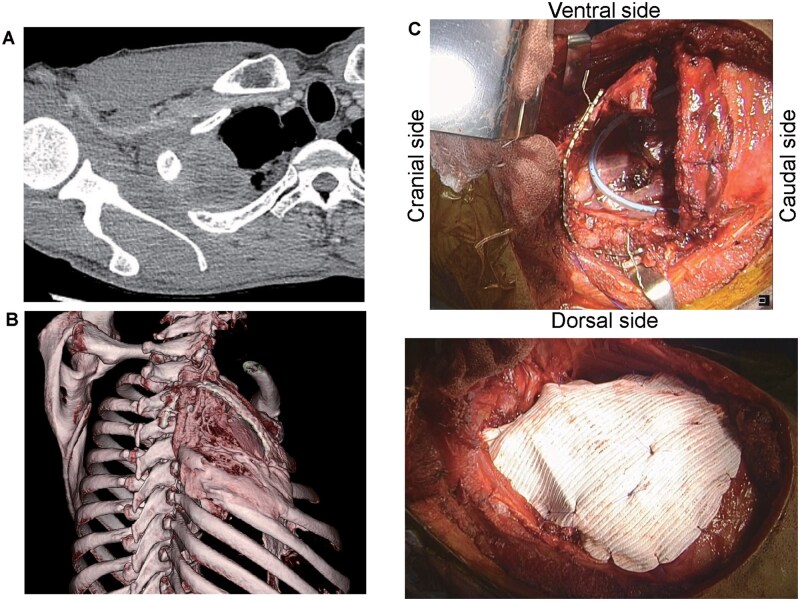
(A) Pre-Treatment Computed Tomography (CT) Revealed a 5-cm Mass in the Right Upper Lobe with Invasion of the First through Third Ribs. (B) Computed tomography (CT) with 3D reconstruction performed after 106 months, demonstrating the reconstructed chest wall (right lateral view). (C) Upper panel: Representative intraoperative view of the reconstruction technique in Case 13. The lateral portions of the first, second, and third ribs were resected en bloc with the right upper lobe. Because the vertebral-side stumps of the second and third ribs were short, placement of a straight plate along the rib axis was difficult. Therefore, an angled plate was used: the short limb was fixed to the vertebral stumps of the second and third ribs with titanium wires, while the long limb was aligned with and fixed to the second rib. Lower panel: A DualMesh was overlaid, with the smooth surface facing the thoracic cavity.
